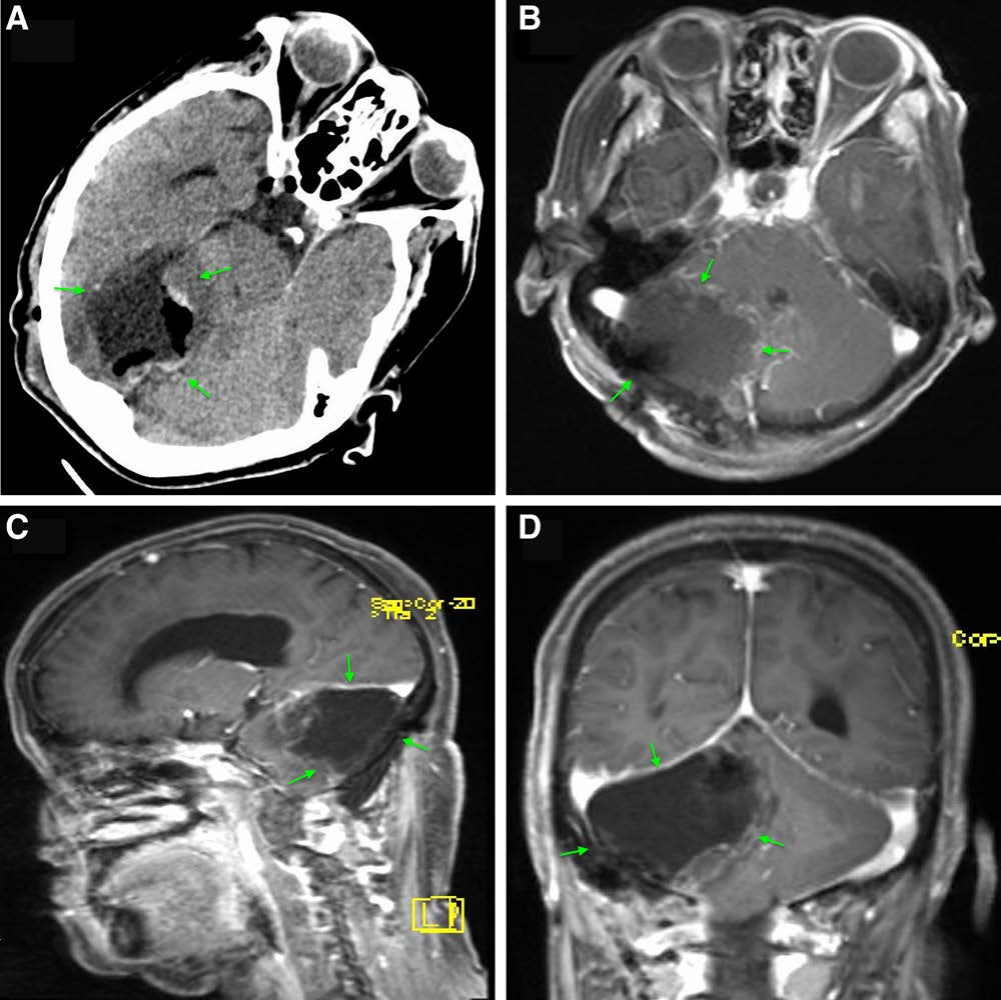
A-D: Are postoperative CT scan and MRIs showing total resection of the lesion. A = CT scan, B = Axial, C = Sagittal, D = Coronal. Green arrows = site of resected lesion.
